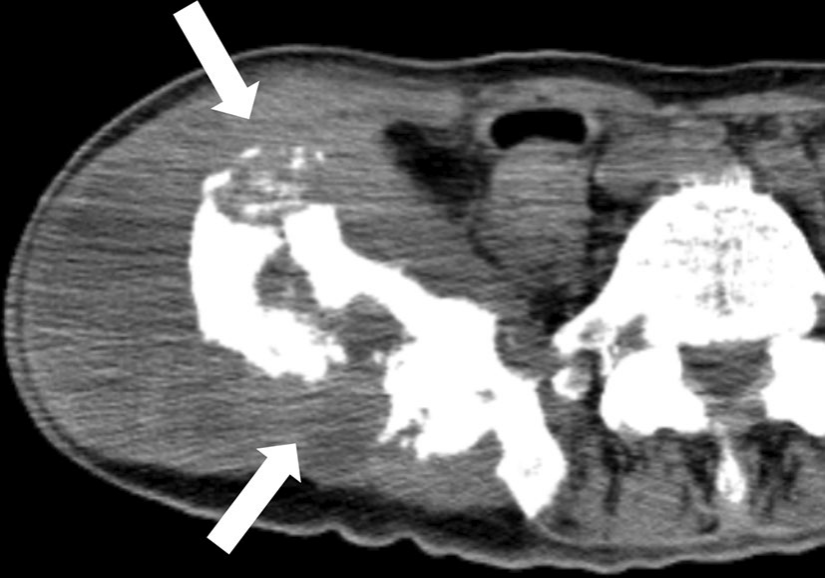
The superiority of postmortem CT for bony evaluation. Easy to understand right iliac metastasis (arrows) of lung cancer before sequential autopsy
